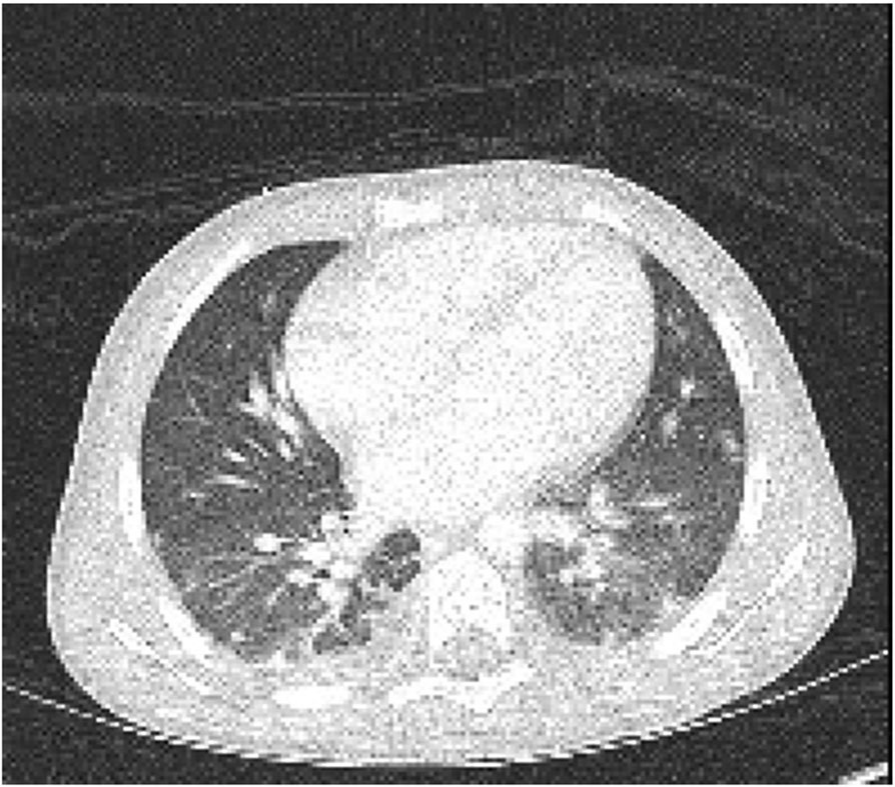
Lung bases captured by chest computed tomography on day 6. Shown in the lungs are numerous peripheral ground glass opacities bilaterally with mild central bronchial wall thickening
